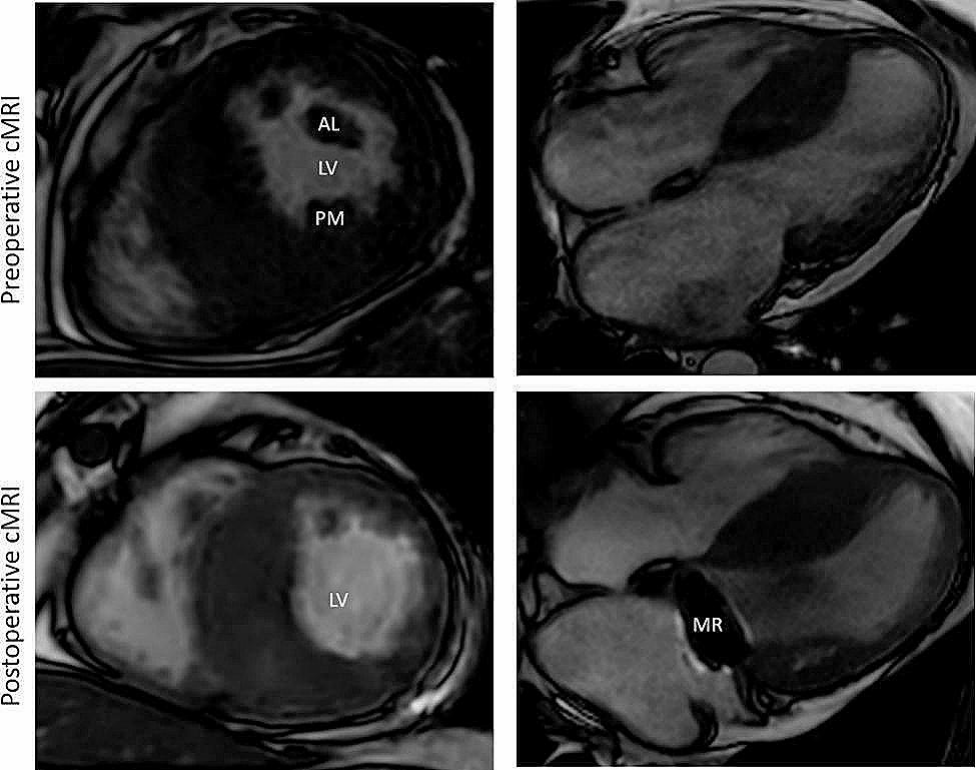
Short axis and 4-chamber view pre- and postoperative cMRI of the hypertrophic obstructive cardiomyopathy. The volume of the LV-cavity after resection of PMs increased. cMRI- cardiac magnetic resonance imaging, AL – anterolateral papillary muscle, PM – posteromedial papillary muscle, MR – mitral valve replacement
